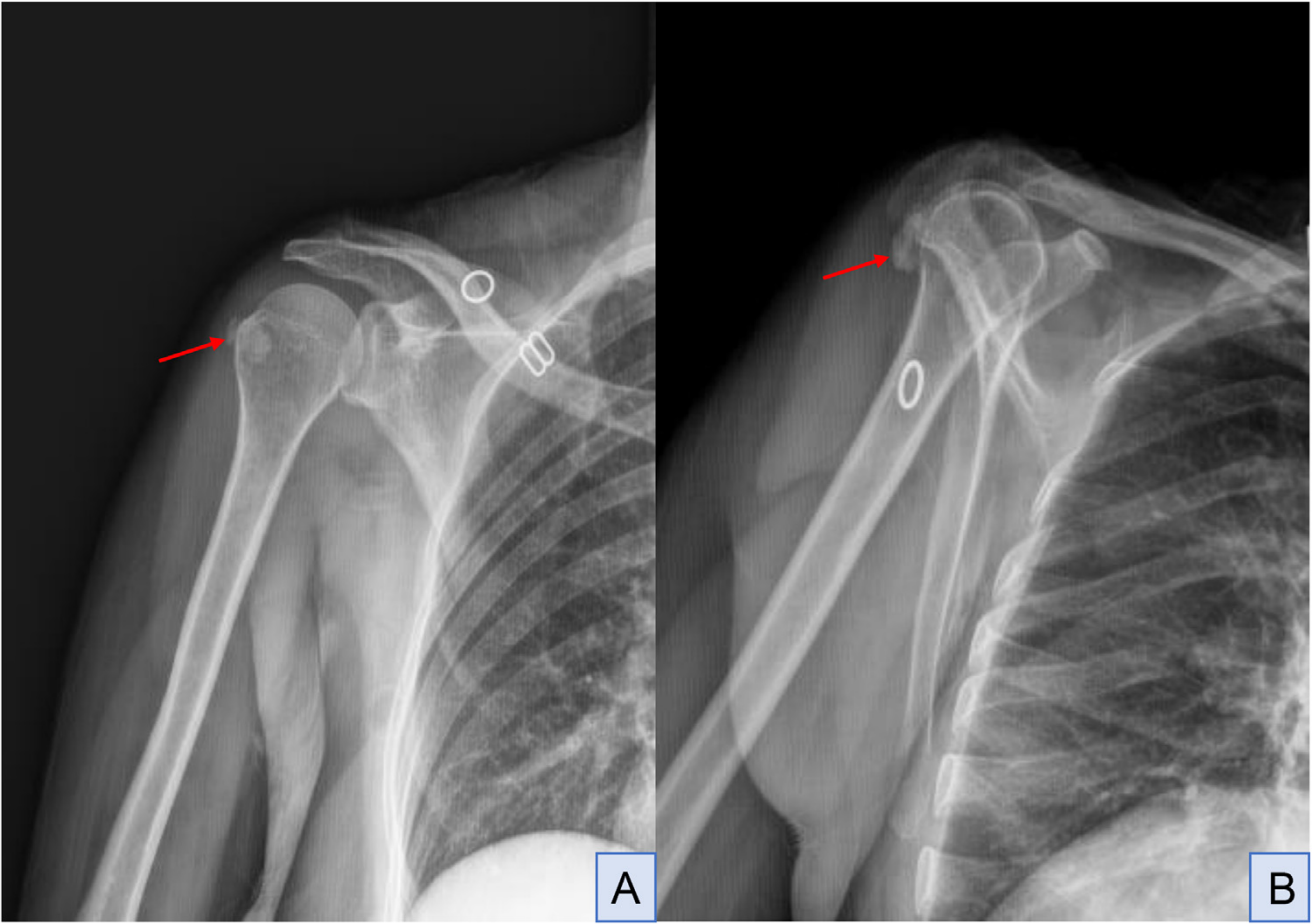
Full-face (A) and internal-rotation (B) radiograph; radiopaque calcifications (red arrow) within the soft tissues adjacent to the greater tuberosity of the humerus.
